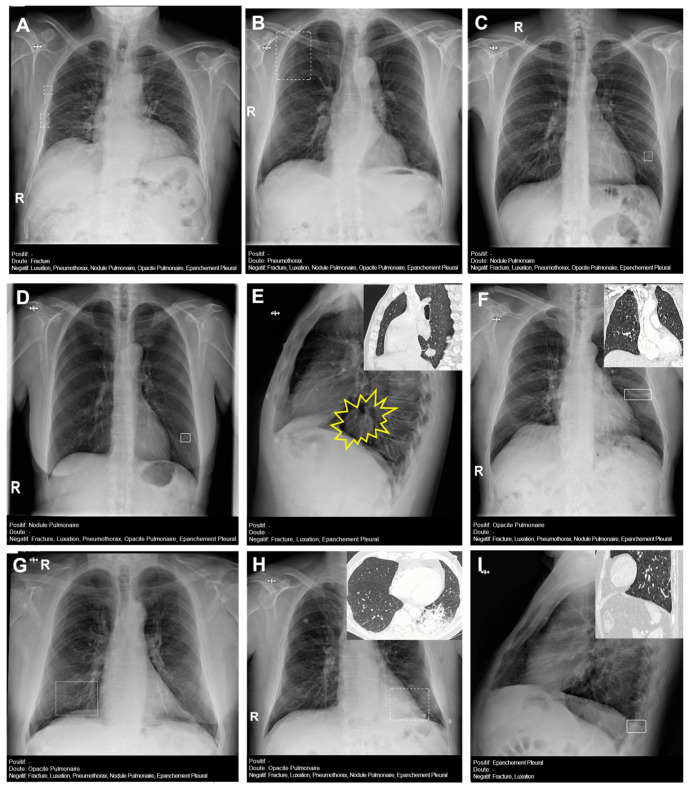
Chest radiographs with AI analysis (and E,F,H,I with the associated sagittal (E,I), coronal (F), and axial (H) CT images). (A) False doubtful right fractures outlined with discontinuous boxes (they are chronic). (B) False doubtful right pneumothorax outlined with a discontinuous box. (C) False doubtful left lower lobe nodule outlined with a discontinuous box. (D) False positive left lower lobe nodule outlined with a continuous box. (E) False negative left lower lobe nodule; there is a nodule (outlined in yellow). (F) False positive left pulmonary opacity outlined with a continuous box (it is a laminar atelectasis). (G) False doubtful right lower lobe opacity outlined with a discontinuous box (it is normal pulmonary vascularization). (H) True doubtful left lower lobe opacity outlined with a discontinuous box (retrocardiac infection). (I) False positive pleural effusion outlined with a continuous box (it is a subtle non-pathological erasure of the posterior costophrenic angle).
